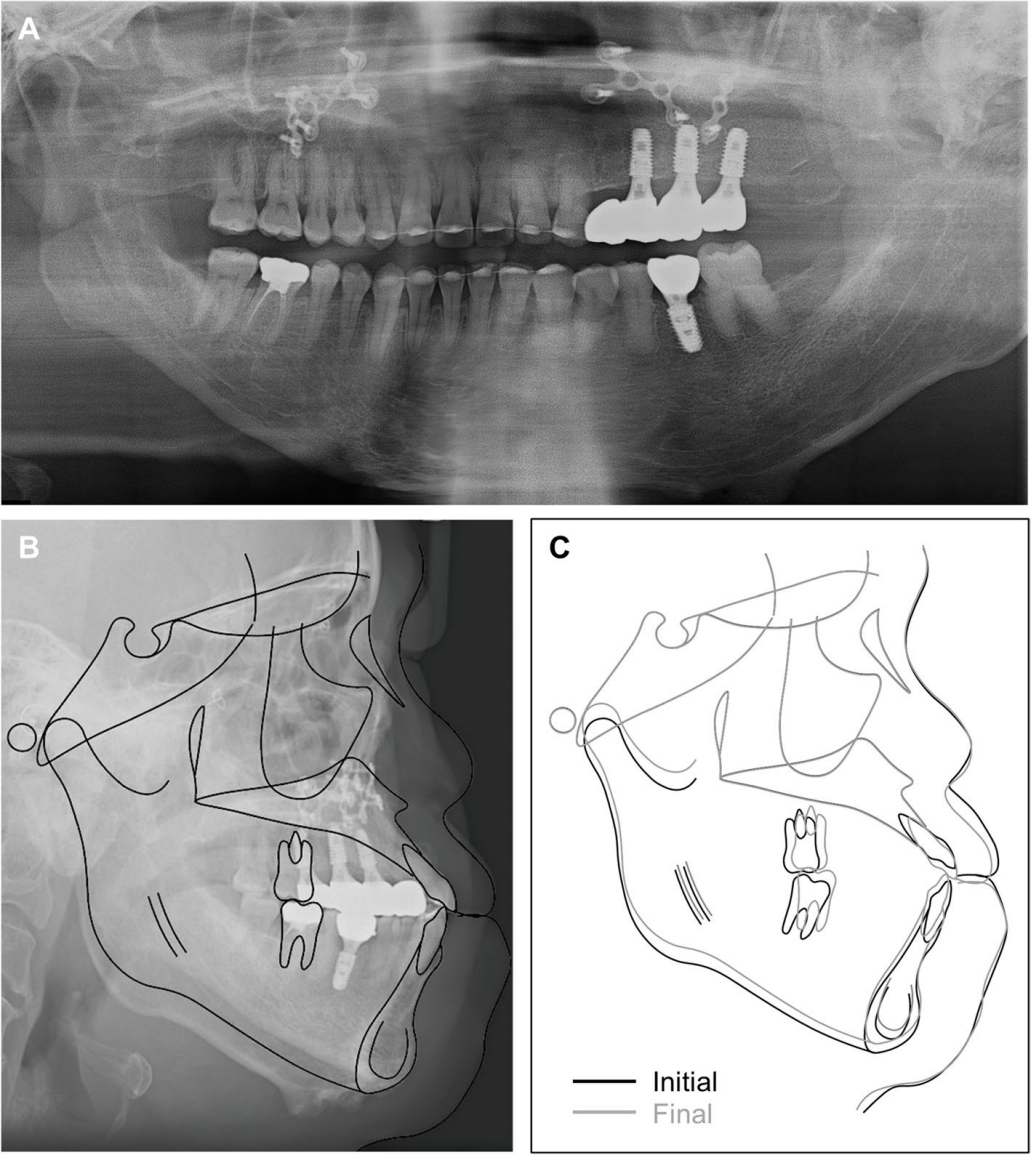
Posttreatment radiographs. A Panoramic radiographs, B Lateral cephalogram and tracing, C Superimposition of initial and final tracing
